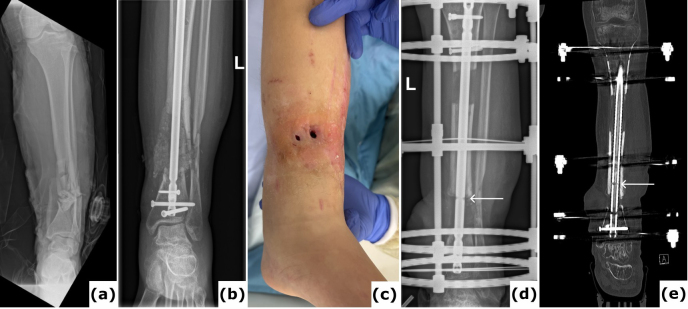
Fracture-related infection (FRI) of the tibia. An 18-year-old patient presented with a Gustilo-Anderson type III open fracture of the tibia and fibula. (a) Anteroposterior radiograph demonstrates a comminuted fracture of both the tibia and the fibula. (b) The anteroposterior radiograph indicates fixation of the fracture using an intramedullary nail. The defect was managed with MIMT. (c) Six months post-injury, the patient was referred with multiple fistulas, confirming FRI. (d–e) Anteroposterior radiograph and computed tomography (CT) images were taken after the revision procedure. Removal of the implant and thorough surgical debridement resulted in a large segmental bone defect. The images show the progression of bone transport over a 10 mm intramedullary nail. White arrows point to the off-label locking hole created during the procedure. Once bone transport is complete, the segment can be secured with a 4 mm interlocking screw and the frame can be removed, which reduces the external fixation time.
